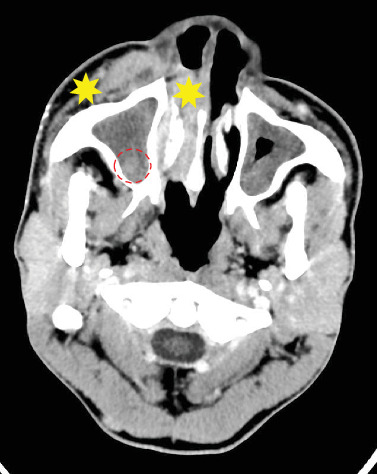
Axial slice of postcontrast CT of the brain and sinuses viewed on soft tissue window, showing facial swelling and postcontrast enhancement of the skin and subcutaneous tissue (yellow star) overlying the right side of the nose, nasolabial fold, and right cheek. An isodense mucosal nodule was observed in the posterior–inferior aspect of the sinus thought to be a polyp (red circle), with postcontrast enhancement and swelling of the inferior and middle nasal turbinates.
